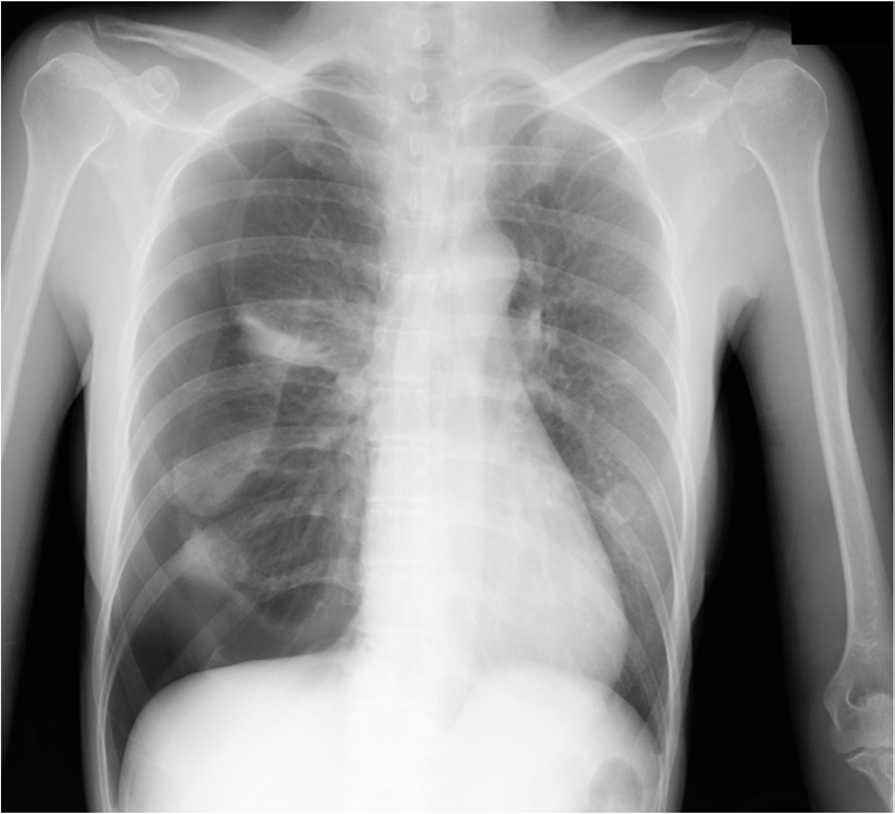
Chest radiography showing right pneumothorax
